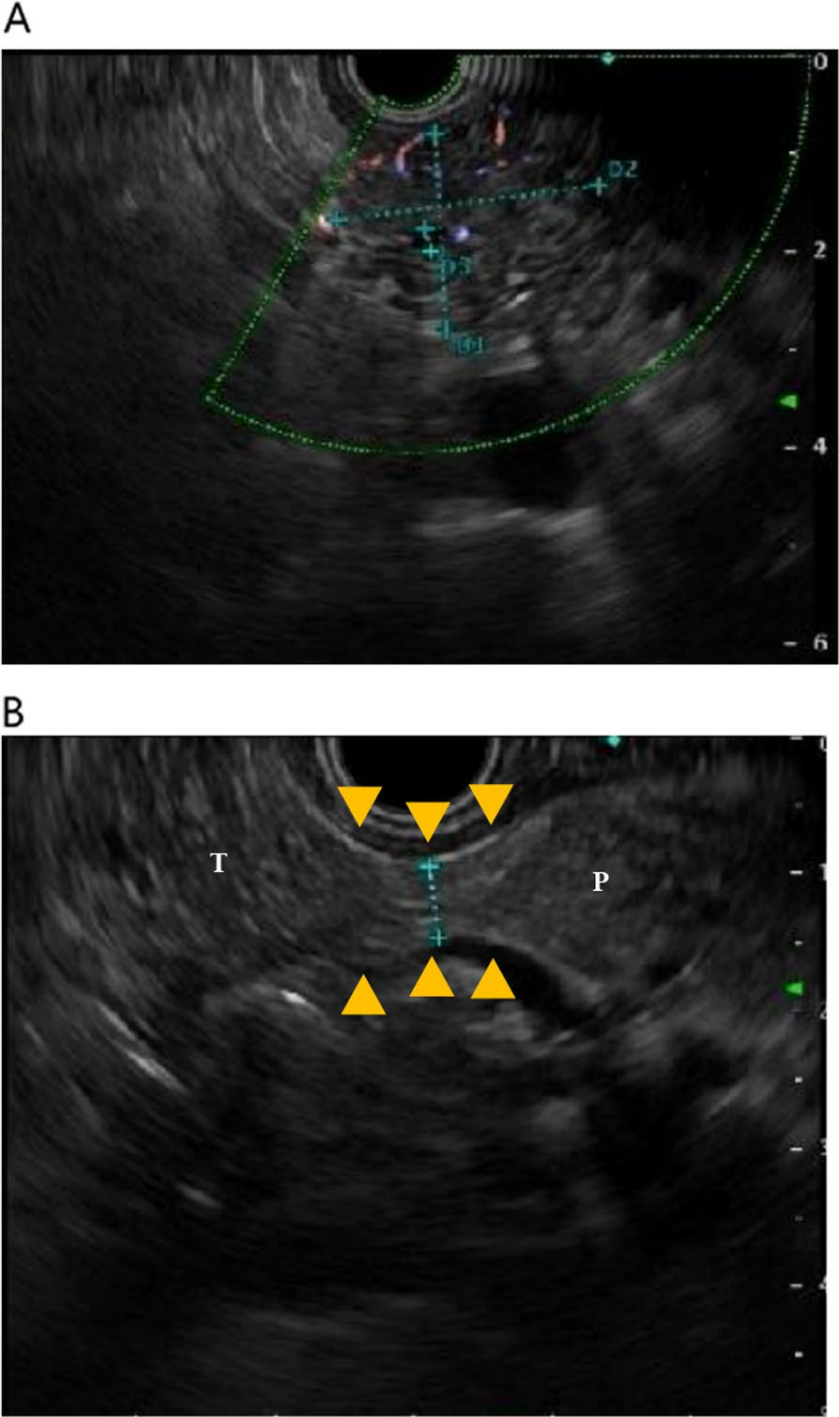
Findings of endoscopic ultrasonography (EUS). A EUS revealed a mass-like lesion in the gastric pylorus with continuity with the gastric wall, which was 26.7 × 20.0 mm in size. B The tumorous lesion (T) was suspected to be continuous with the pancreatic parenchyma (P) with a partial thickness of approximately 5 mm (yellow arrow)
